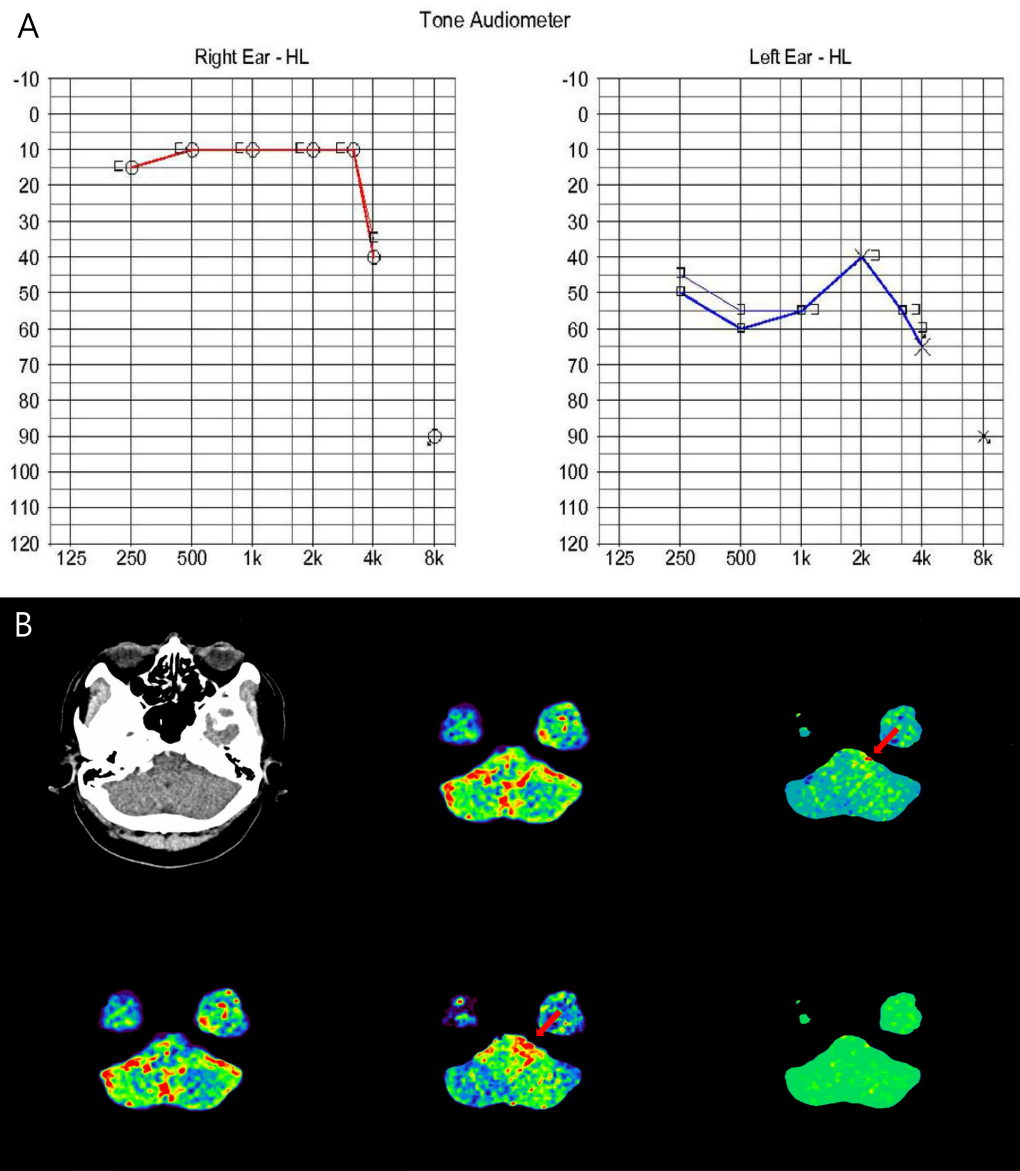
Pure-tone audiogram 2 months later (a) documented persistent unilateral sensorineural, pure-tone average of 53 dB in the left ear. Additionally, perfusion CT (b) showed that the perfusion deficits remained in the left cerebellum along the PICA and AICA territories (red arrow)
